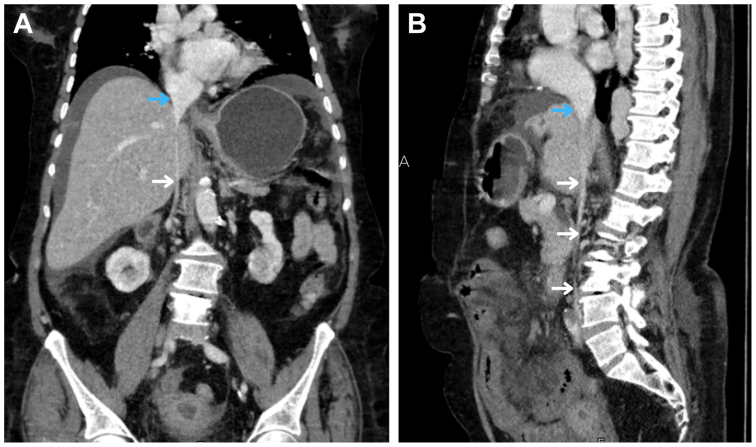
Contrast-enhanced abdominal computed tomography angiography (CTA) images in coronal (A) and sagittal (B) planes demonstrate a normal-caliber supra-hepatic inferior vena cava (IVC; *blue arrows*) followed by small-caliber but patent infra-hepatic IVC (*white arrows*). On the sagittal plane (B), the entire length of the IVC is visualized extending from its origin at the iliac bifurcation to its entry into the right atrium.
